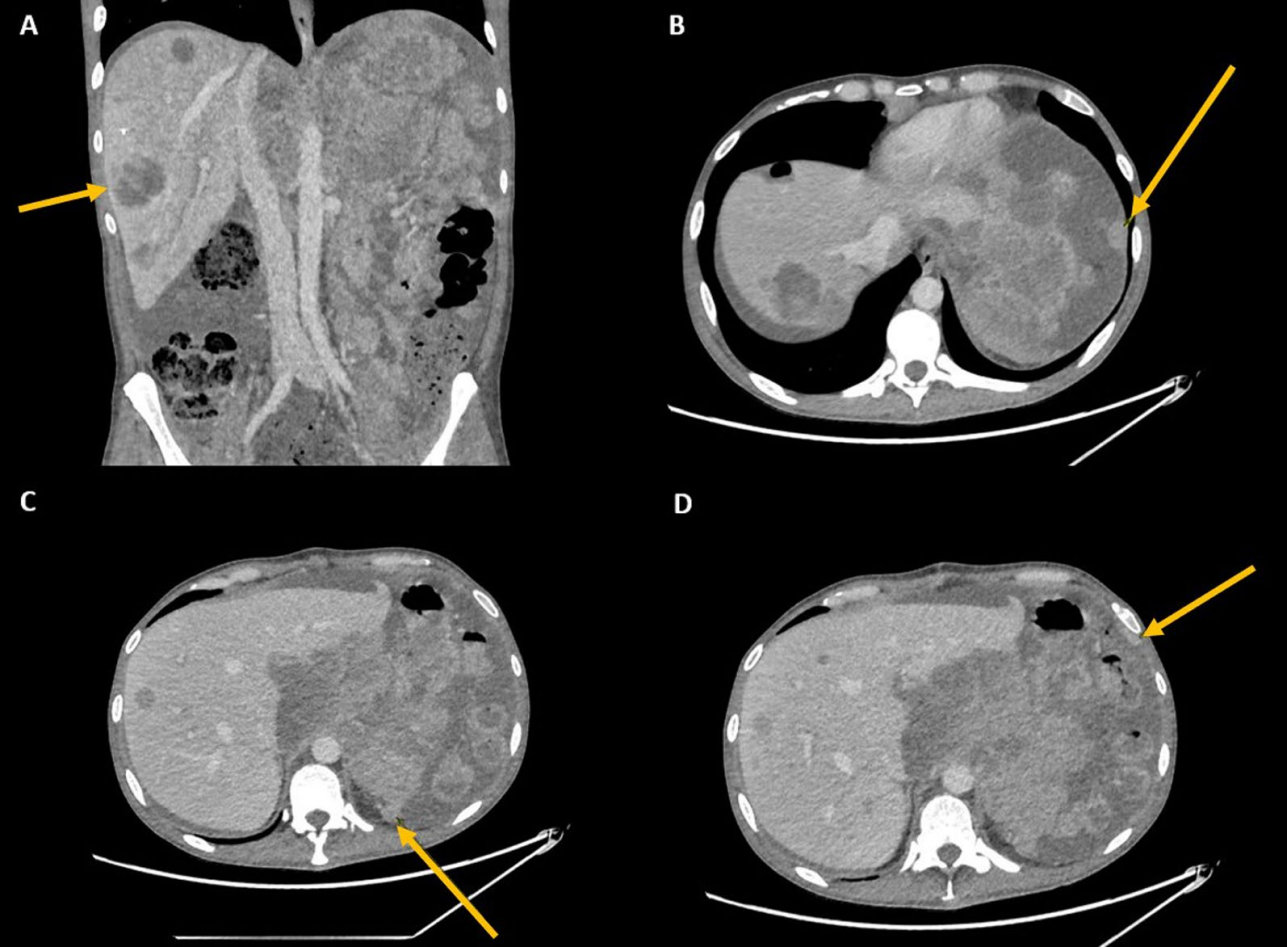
CT images demonstrating A extensive hepatic metastasis, B left subdiaphragmatic deposits, C splenic bed deposits and D parietal peritoneal deposits
